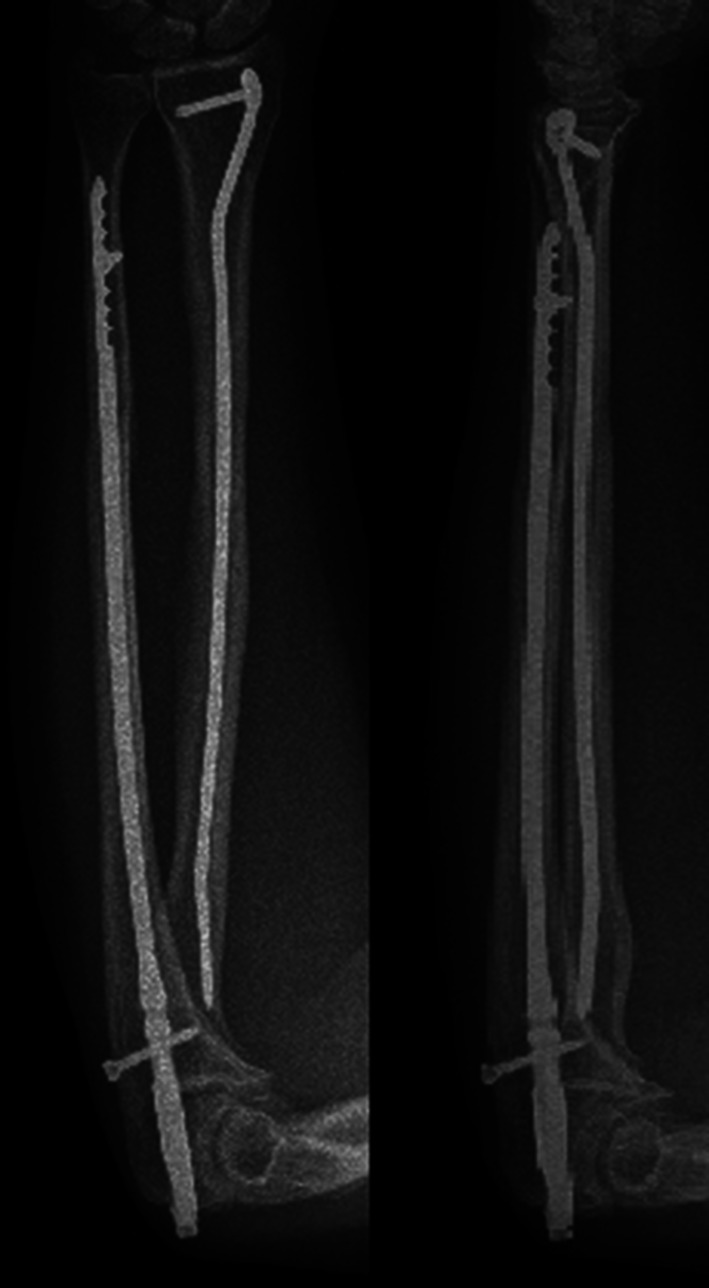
AP and lateral radiograph showing complete union of the radius and ulna fracture after 3 months of surgery
